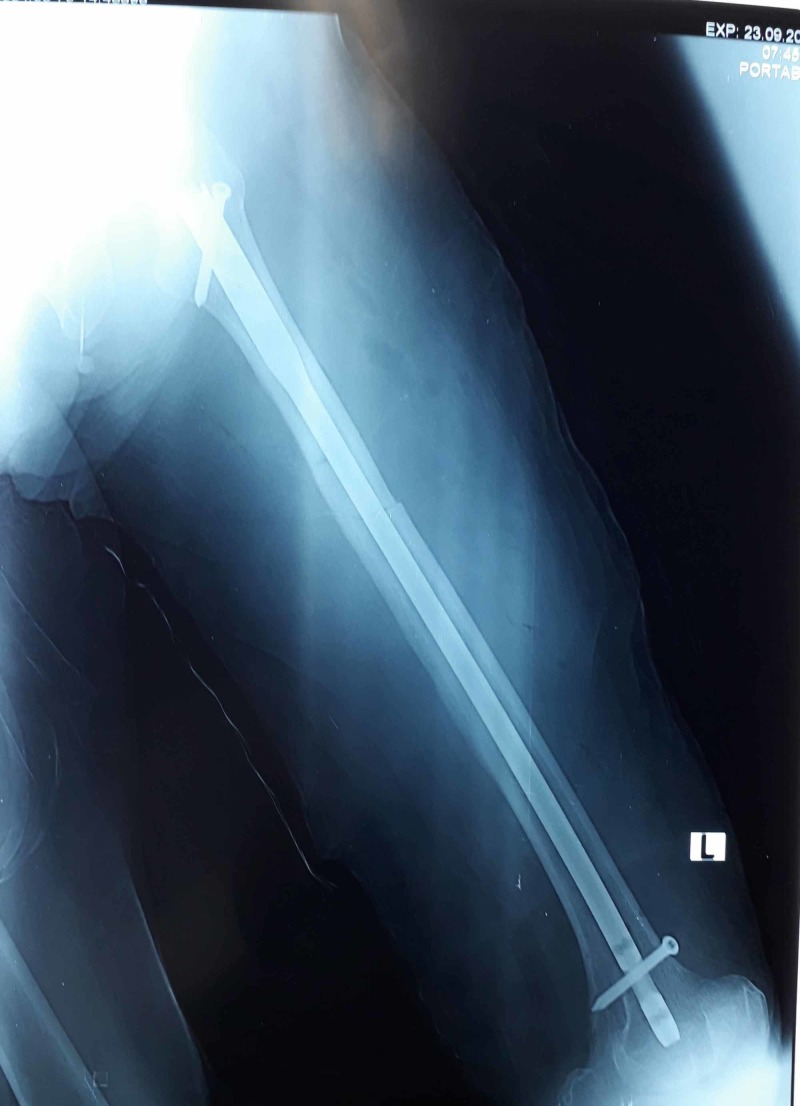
Postoperative X-ray of patient showing fixation with intramedullary interlocking nail
